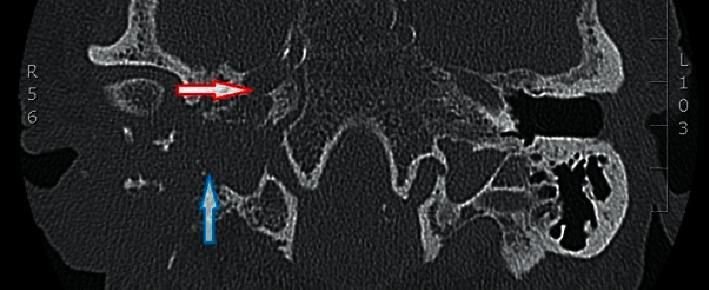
Axial view of temporal CT 5 months after the initial surgery showing complete opacification and massive bone erosion of the middle ear and mastoid on the right side indicating an extended soft tissue mass adjacent to the carotid canal (red arrow) and jugular bulb (blue arrow) and partially obliterating the temporal mandibular joint.
